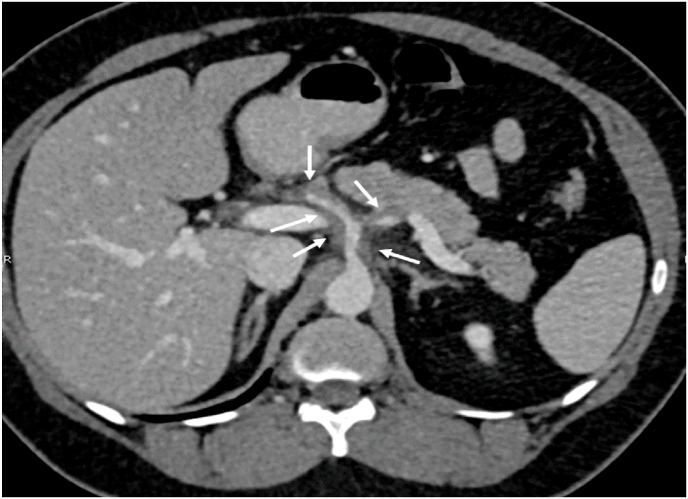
Axial contrast-enhanced CT image acquired at the level of the celiac artery showing circumferential thickening of the wall of the celiac, splenic, and common hepatic arteries (white arrows) with mural enhancement indicating inflammation.
